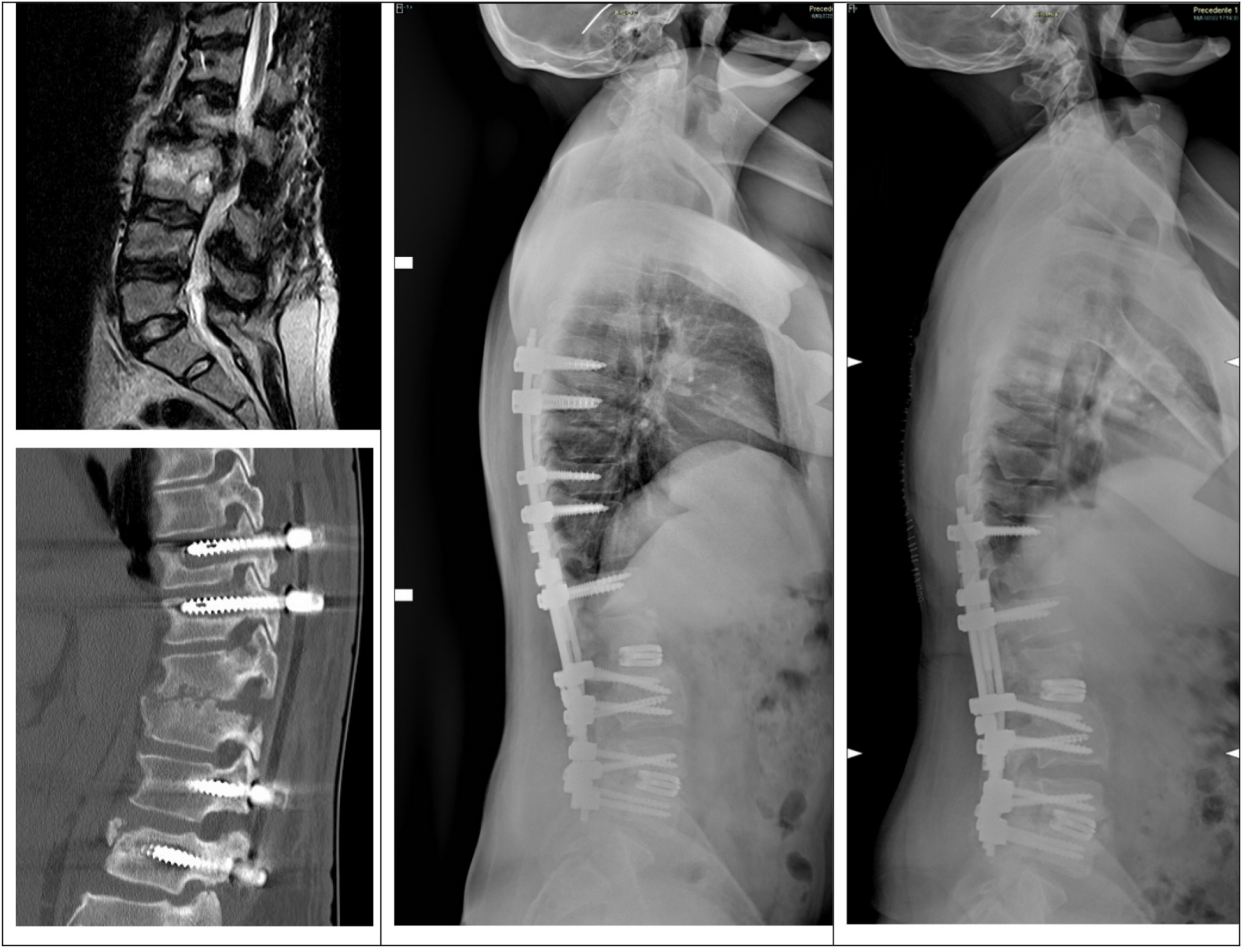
Top-left, preoperative MRI with signs of spondylodiscitis and stenosis. Bottom-left, CT showing mobilization of the screws after revision and extension T6–L2. Center, postoperative x-ray after the revision and extension of the instrumentation T6–L5 with expandable cages in L1–L2 and L4–L5. Right, postoperative x-ray after revision with shortening of the instrumentation to T10–L5.
